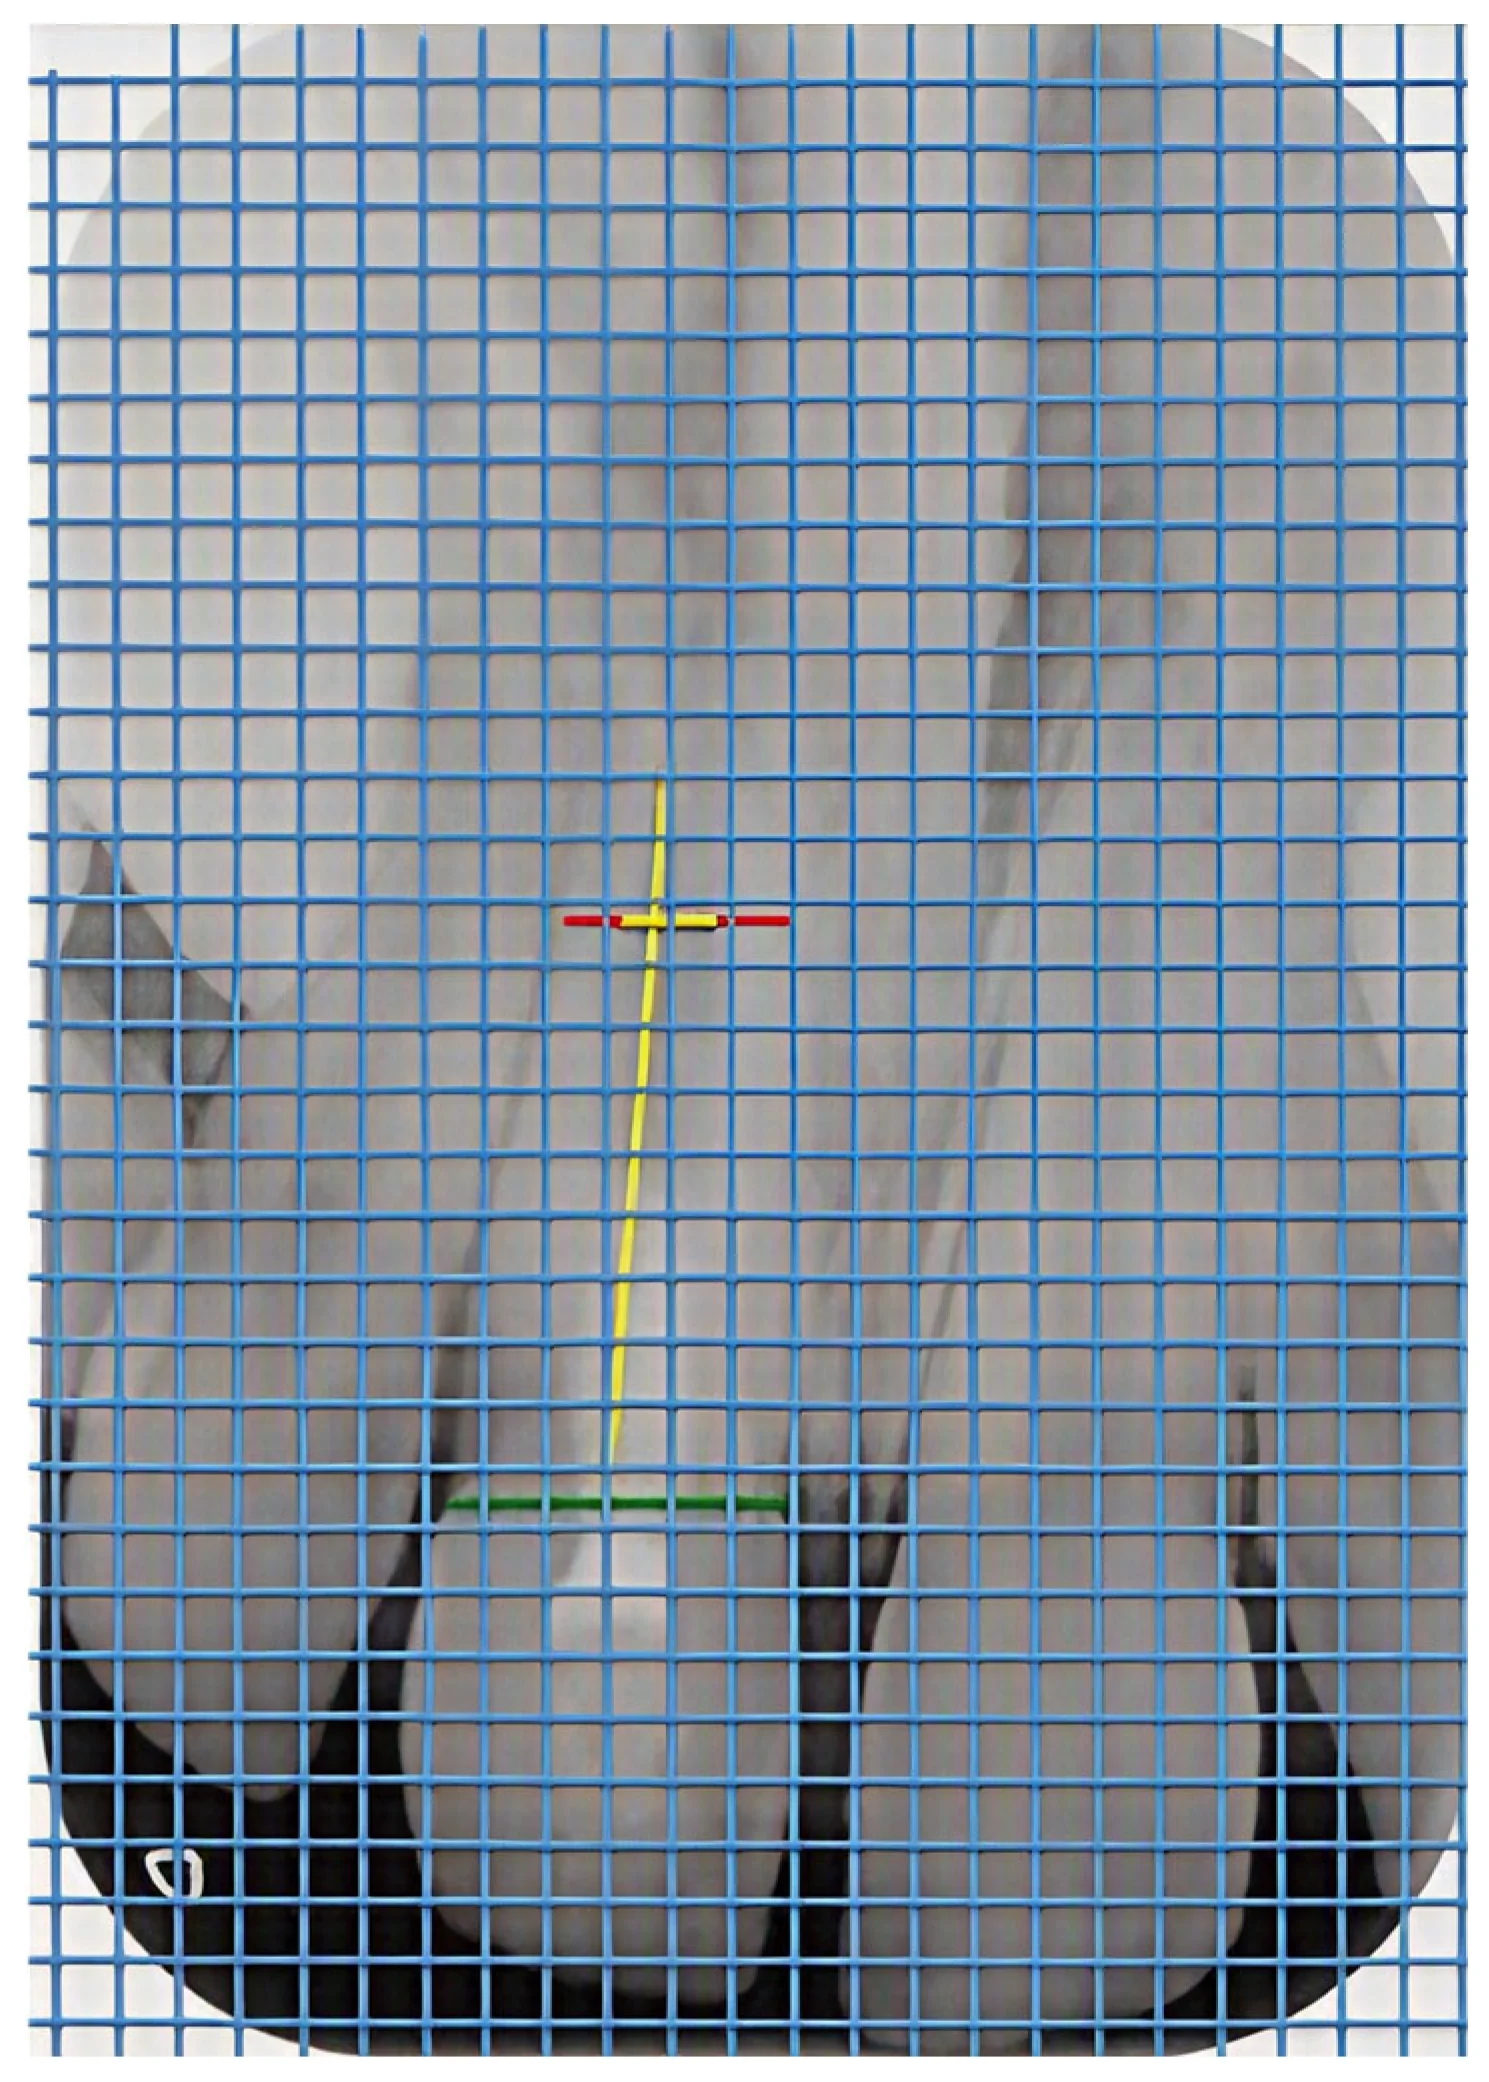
Measurement of the differences in root length and dentin wall thickness from the radiographs. The vertical yellow line denotes the distance from the cementoenamel junction (CEJ) to the radiographic apex of the tooth. The horizontal yellow line represents the distance between the internal dentinal walls at the apex, the red line measures the distance between the external dentinal walls at the apex, while the green line denotes the same measurement at the CEJ.
